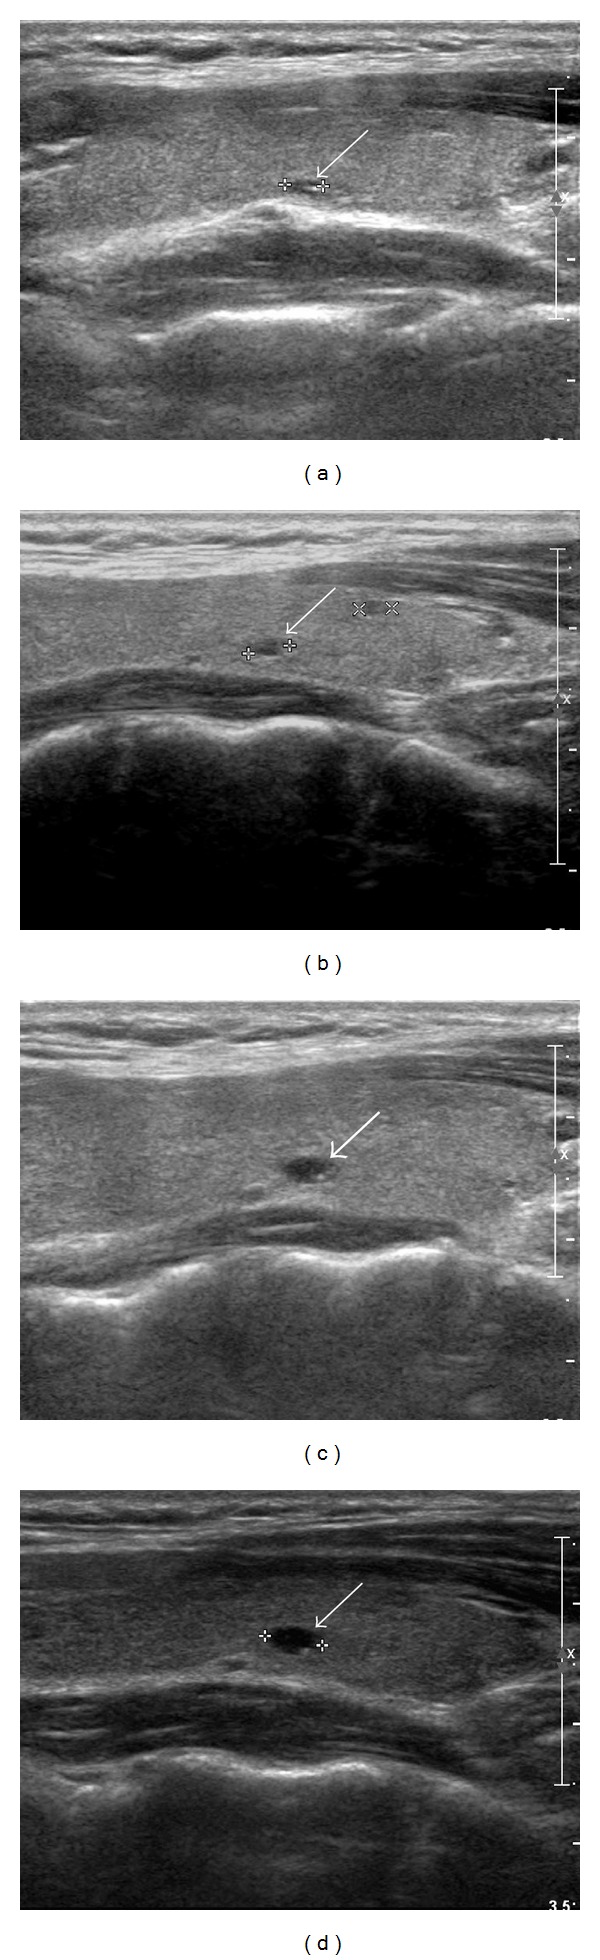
A 27-year-old woman with gradual increase in a thyroid colloid cyst. Preoperative longitudinal sonogram (a) shows a colloid cyst with an anechoic nodule and a comet-tail artifact in the left thyroid lobe (arrow, 3.2 mm at its largest diameter). Upon sequential US follow-ups, this colloid cyst demonstrates a gradual increase at a 1-year (b) (arrow, 3.5 mm at its largest diameter), a 3-year (c) (arrow, 4.7 mm at its largest diameter), and a 5-year (d) (arrow, 5.1 mm at its largest diameter) postlobectomy.
